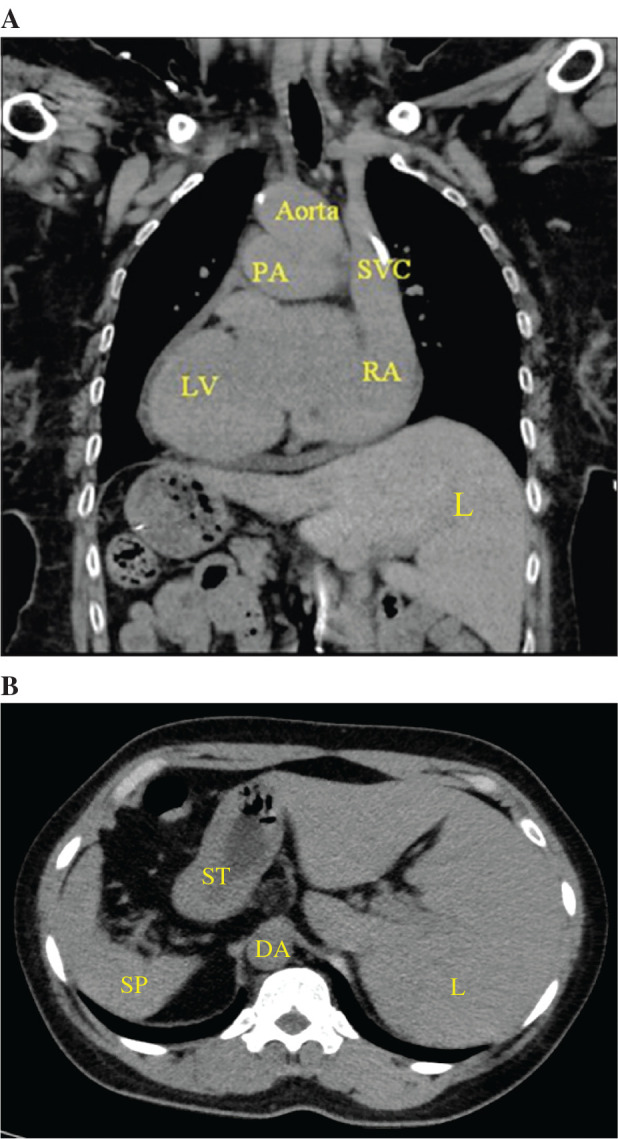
A: Computed tomography of the chest revealed dextrocardia with superior vena cava connected to the right atrium in the left-sided mediastinum. The aortic arch was rotated to the right-sided posterior mediastinum. B: Reversal of the normal anatomy in situs inversus is shown in the abdominal view. *Abbreviations*: DA, descending aorta; L, liver; LV, left ventricle; PA, pulmonary artery; SP, spleen; ST, stomach; SVC, superior vena cava.
